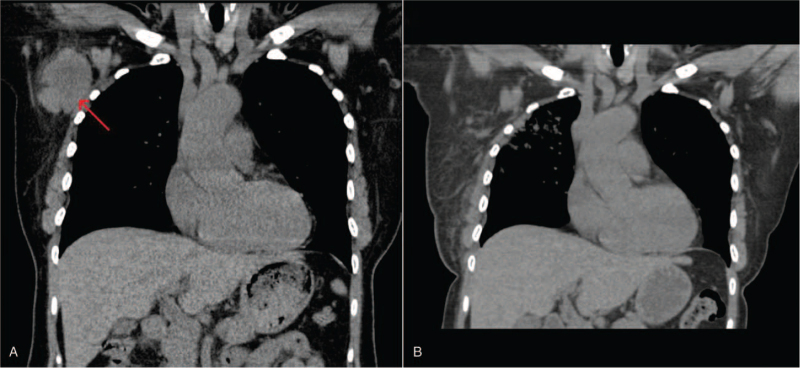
Near-complete radiographic response in right axillary lymph node conglomerate (red arrow) seen on CT scan prior to radiotherapy (A), and on subsequent CT scan 4 months after completion of right axillary radiotherapy (4500 cGy in 15 fractions) and initiation of paclitaxel chemotherapy (B).
